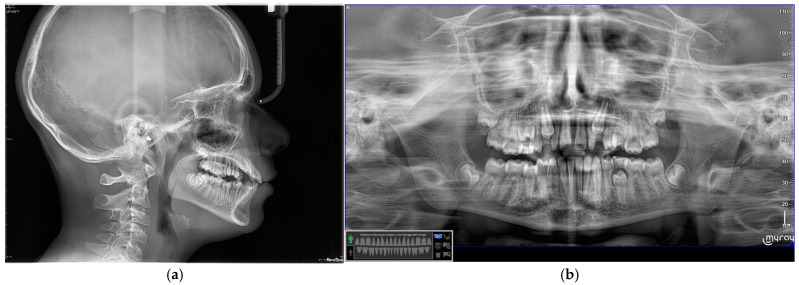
(a,b) Radiographic examination.
